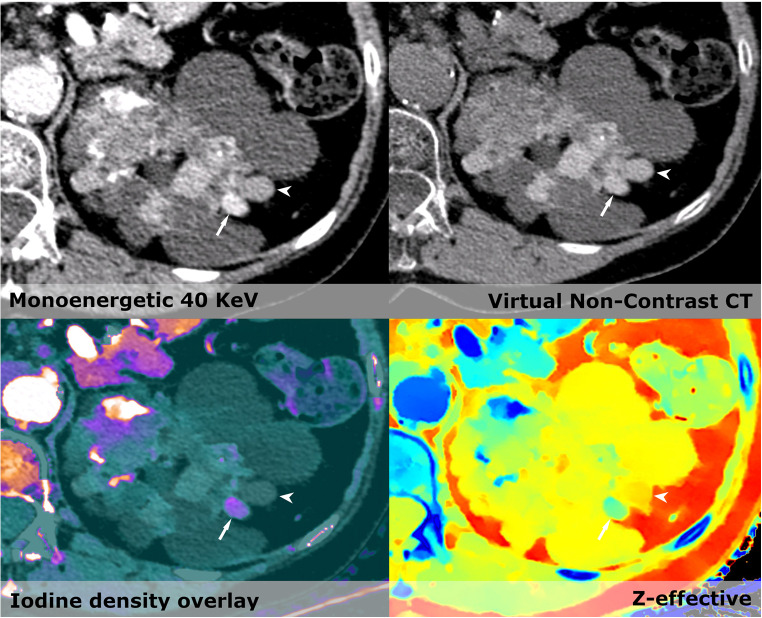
Conventional CT shows several hyperdense lesions within the kidney besides the simply cysts. VNC shows differences in several areas, most markedly within one of the two hyperdense lesions (Arrow) in the lateral part of the kidney. Iodine density overlay and Z-effective show a clear enhancement in the area mentioned. Next to this is a hyperdense cyst (arrowhead) with no enhancement and Z-effective values below 8. The diagnosis are most likely renal carcinoma within a polycystic kidney
